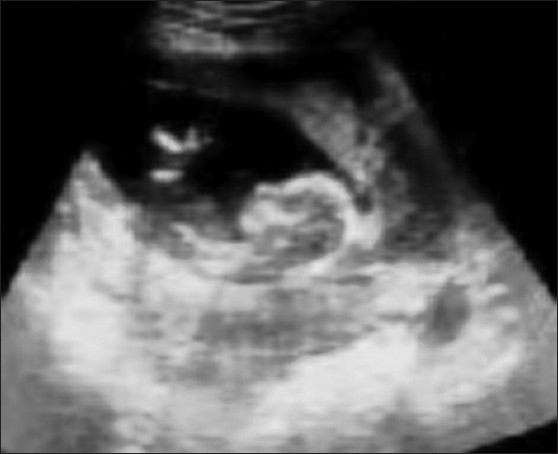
Ultrasonography showing intra-abdominal pregnancy
